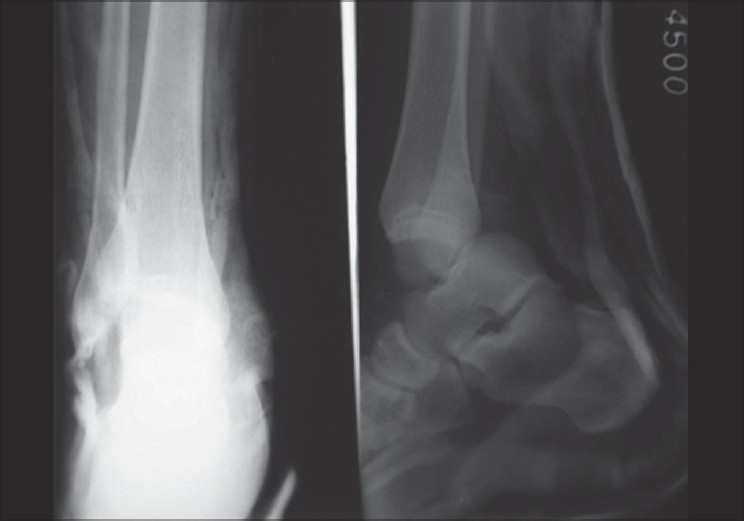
AP and lateral X-ray of right ankle shows posterior dislocation of the ankle
